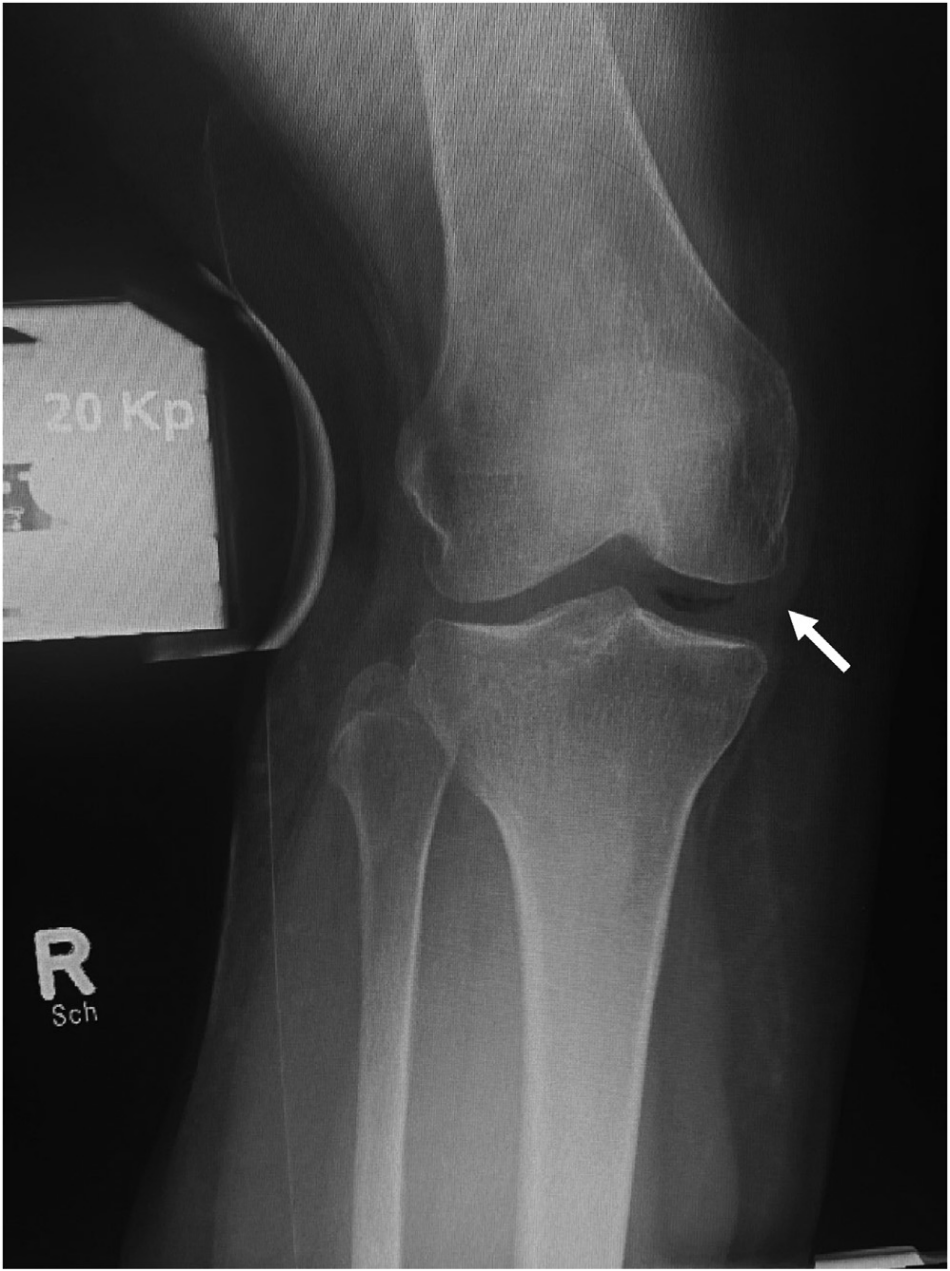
Typical valgus stress radiograph with a medial joint opening of 12 mm.
